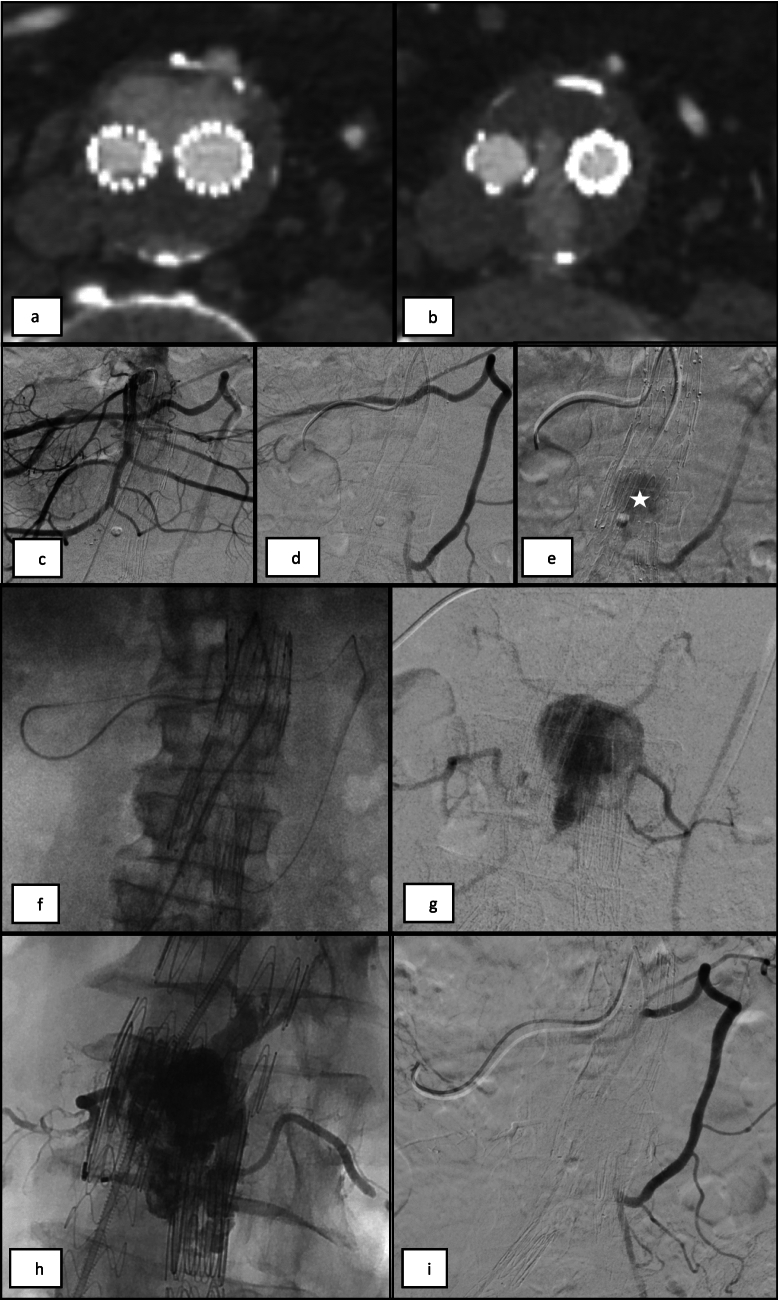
Transarterial embolisation of an IMA T2EL. (a, b) CTA imaging demonstrates a T2EL arising from the IMA with contrast filling the aneurysm sac anteriorly and extending between the iliac limb endografts. (c-e) Selective angiogram from the middle colic artery of the SMA opacifies hypertrophied arc of Riolan. IMA and endoleak nidus within the aneurysm sac (*). (f) A 2.3Fr microcatheter was advanced through the arc of Riolan and IMA origin, into the aneurysm sac. (g) Angiogram from the aneurysm sac opacifies the EL nidus and multiple lumbar outflow branches. (h) Embolisation of T2EL with NBCA injected via the microcatheter spreads into the sac, lumbar vessels and IMA. (i) Completion angiogram demonstrates no further endoleak
